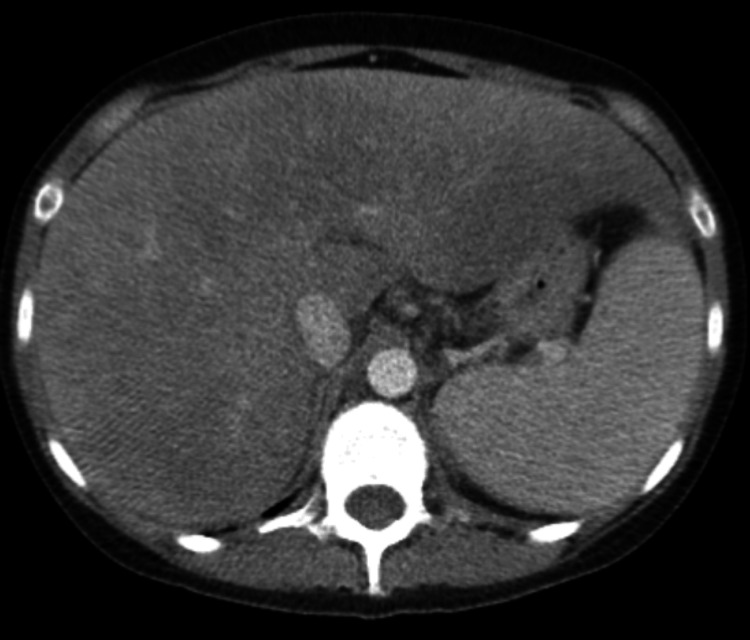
Axial view of computed tomography (CT) of abdomen showing hepatomegaly and splenomegaly.
